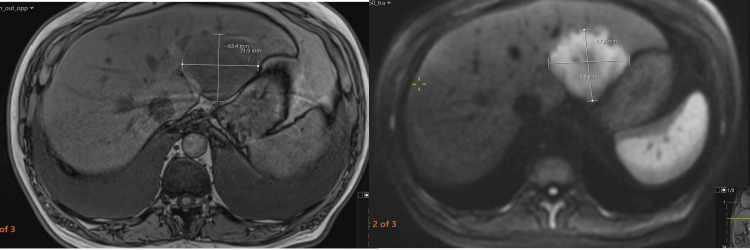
MRI T1 fat-saturated (left) and diffusion-weighted image (right) Arrows indicate a liver mass (left panel) and strong diffusion restriction on diffusion-weighted image (right panel).
